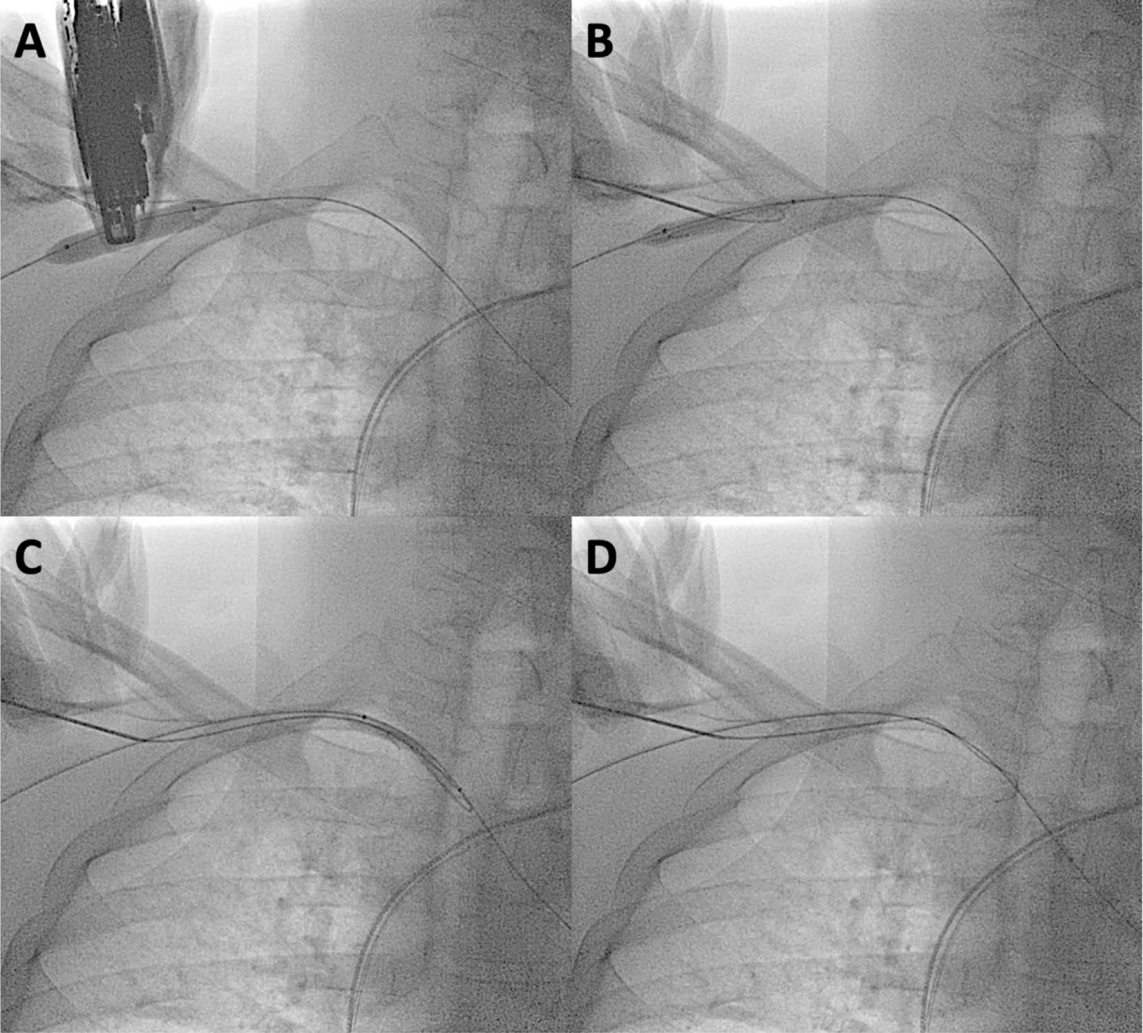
The puncture technique "On the Balloon". (A) The peripheral balloon is inserted through radial access and positioned at the puncture site. Subsequently, the artery is punctured together with the balloon under ultrasound guidance. (B) The guidewire is inserted into the balloon. (C) The guidewire and balloon are jointly advanced to the aorta. (D) The guidewire is removed from the balloon and advanced back to the aorta (afterward, the balloon is removed). Refer to supplementary Video S1.
